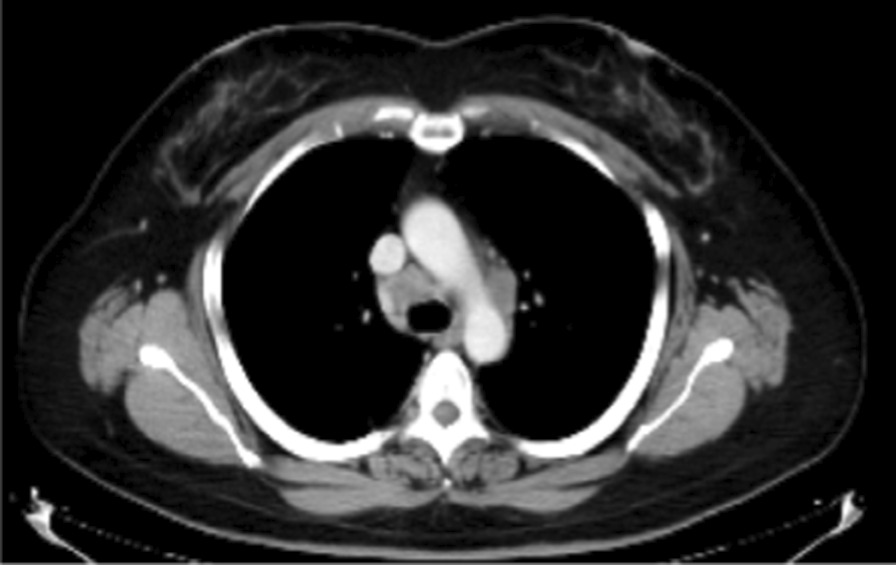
Thoracic computed tomography with contrast enhancement at 2 weeks after administration of steroid showing reduced mediastinal lymph nodes in size
